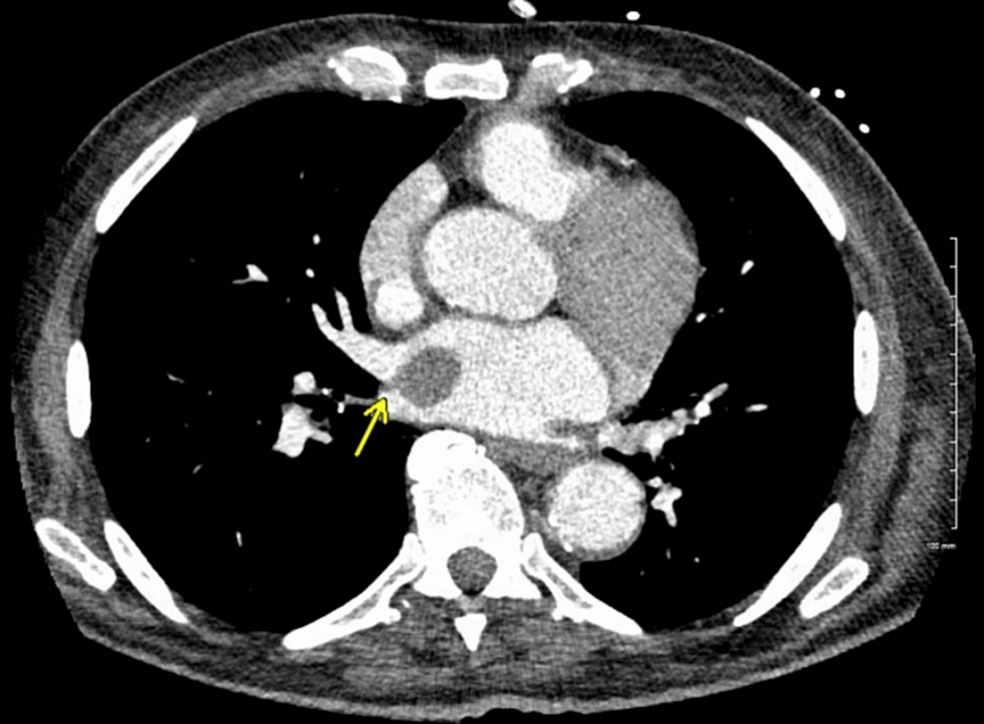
CT image showing lobulated circumscribed mass (yellow arrow) attached to the right lateral wall of the left atrium. CT, computed tomography
